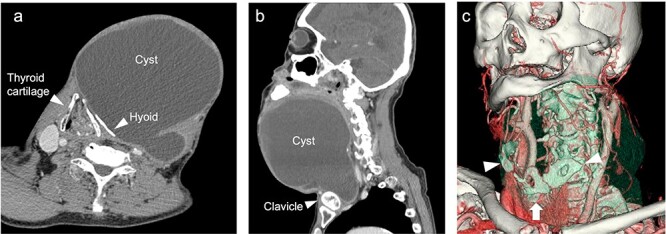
CT scan imaging of the cyst (a, b). The thyroid cartilage pressed toward the right side and dislocation of the hyoid bone (c) 3D-imaging of CT scan. Deformed hyoid bone (arrowhead) and pressed thyroid cartilage (arrow).
